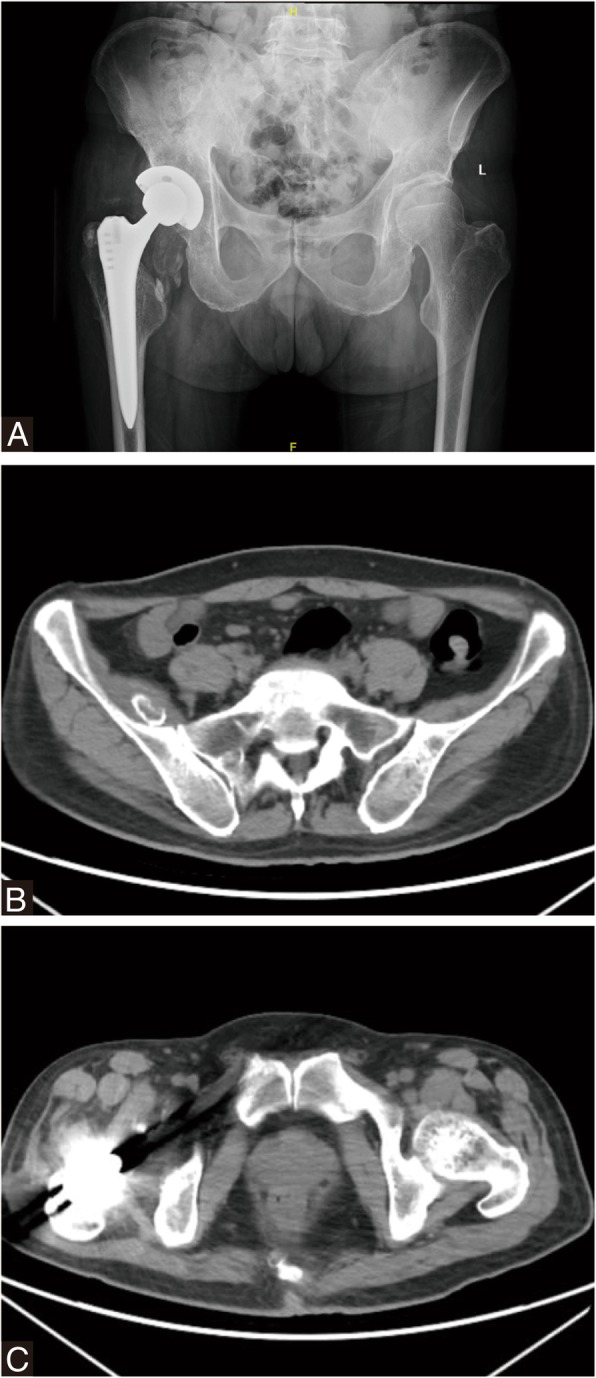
Two months following-up after re-revision surgery. a Radiograph showing unremarkable pseudotumor surrounding hip and mass in pelvis. b-c A transaxial view of the CT showed a capsule wall in pelvis and lower density shadow surrounding the right hip
